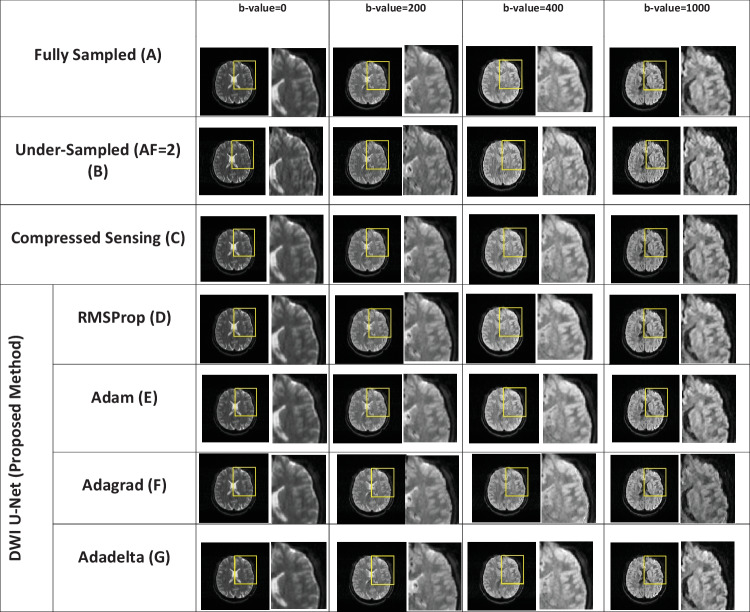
Reconstructed images of the human head multidirectional dataset for the proposed method (DWI U-Net) with different optimizers, i.e., RMSProp, Adam, Adagrad, Adadelta, and Compressed Sensing at *AF* = 2 with different *b*-values, i.e., 0, 200, 400, and 1000 s/mm.^2^ (left to right in each column)
